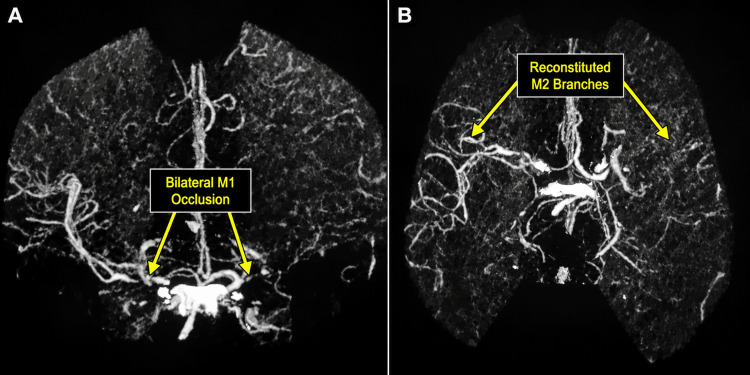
Computed tomography angiography, maximum-intensity-projection (MIP) reconstructions (A) Coronal MIP shows bilateral M1 segment occlusion, with arrows indicating the sites of the absent M1 segments at the internal carotid artery bifurcations. Attenuated anterior cerebral arteries are reconstituted via collateral pathways, and a fine network of hypertrophied lenticulostriate and basal perforating collateral vessels is present in the deep basal ganglia bilaterally, producing the characteristic puff of smoke appearance; the basilar artery and posterior circulation remain well opacified; (B) Axial MIP at a more superior level demonstrates reconstituted M2 branches (arrows), relatively preserved posterior cerebral arteries, a prominent basal collateral network, and markedly reduced cortical opacification in the bilateral middle cerebral artery territories, with faint peripheral vessels suggesting leptomeningeal collateral reconstitution. These findings indicate chronic bilateral steno-occlusive disease with extensive collateral formation rather than an acute, thrombectomy-eligible large vessel occlusion, and are suggestive of a moyamoya vasculopathy; they are not independently definitive, and DSA remains the reference standard for confirmation.
